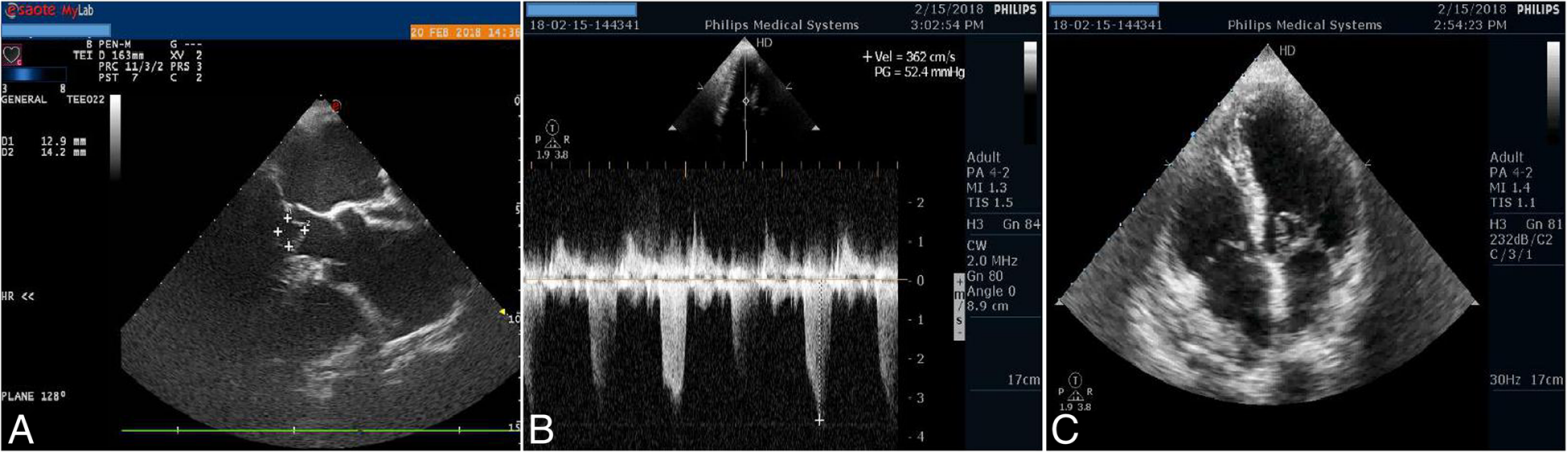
a Transesophageal echocardiography demonstrated tissue dimensions and location. b Transthoracic echocardiography pressure peak gradient measured by continuous wave Doppler across left ventricular outflow tract. c Transthoracic echocardiography apical four-chamber view showed the relation between the accessory mitral valve tissue and the anterior leaflet of the mitral valve
